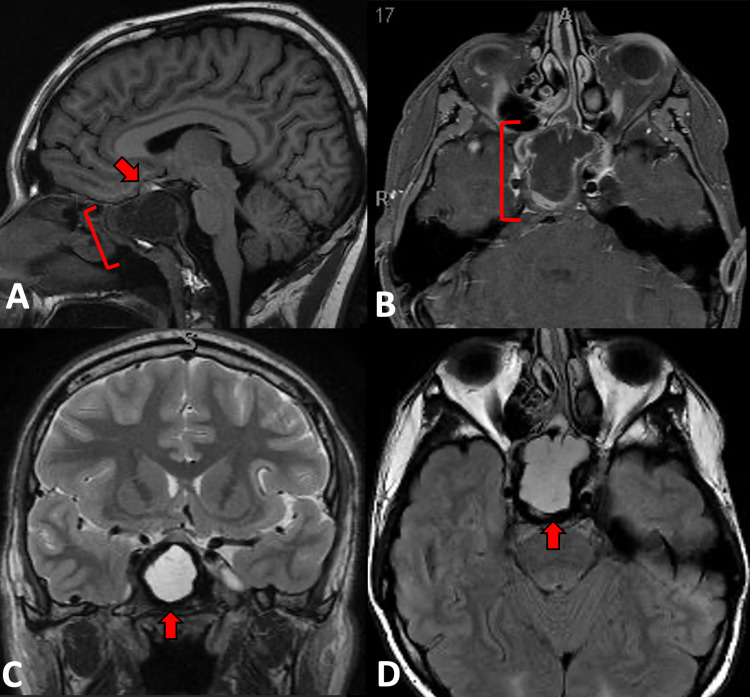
MRI Sagittal T1 (A) and axial (B) T1 post-contrast images reveal a hypointense partially septated expansile mass expanding the sphenoid sinus. Superior pituitary gland displacement (arrow on image A) can be appreciated. The mass has enhancing, nodular sidewalls (bracket on image B). Coronal T2 (arrow on image C) and FLAIR (arrow on image D) images show a hyperintense, multilobulated expansile lesion without fluid-fluid levels. FLAIR - fluid-attenuated inversion recovery
